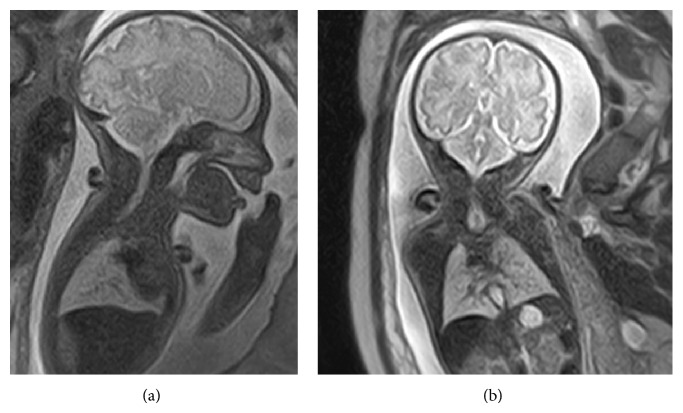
Case report: fetal MRI. Saggittal (a) and axial (b) view. Absence of continuity between subglottic area and bronchial bifurcation: Floyd's classification of type II.
